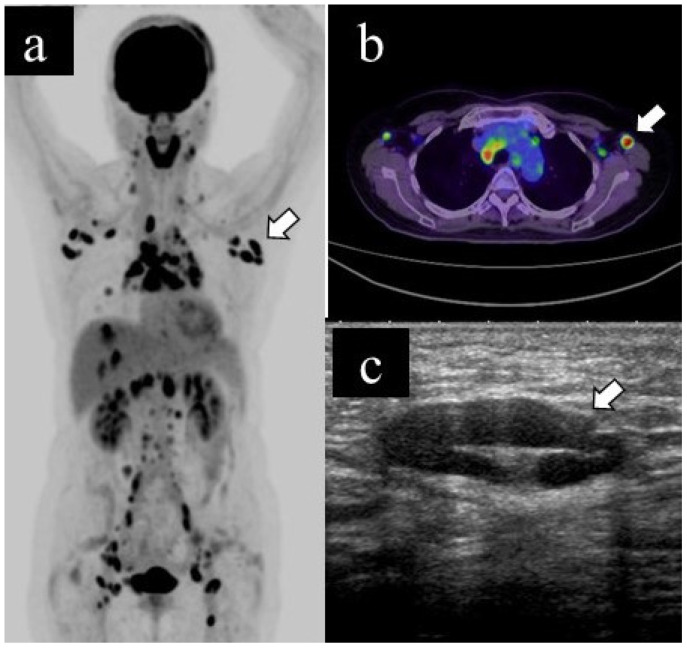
Representative case of benign lymphadenopathy. (a) Maximum-intensity projection (MIP), (b) transaxial 18F-FDG PET/CT image and (c) US image of benign lymphadenopathy.
